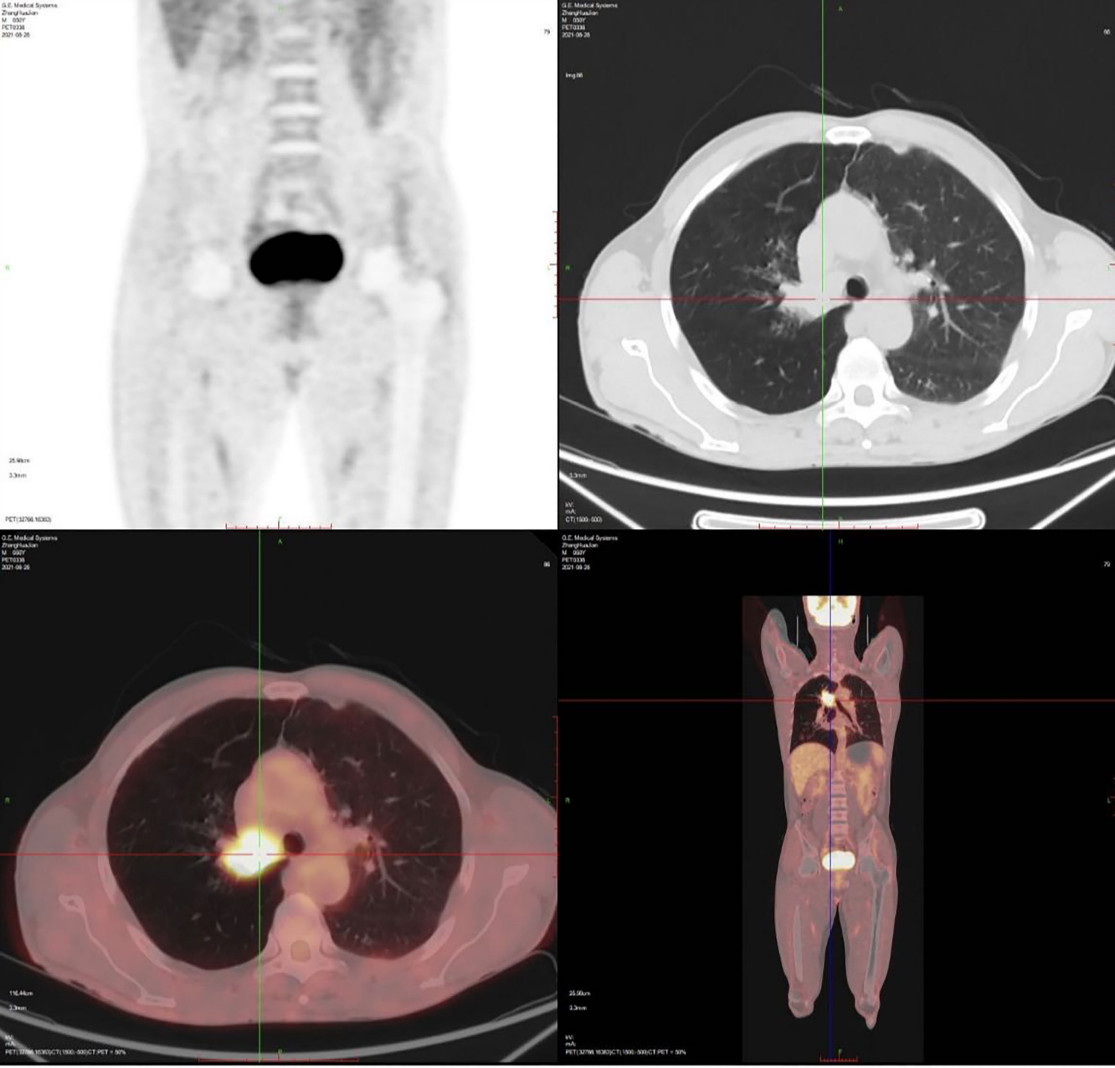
PET-CT showed there were only isolated lung lesions in the whole body.
